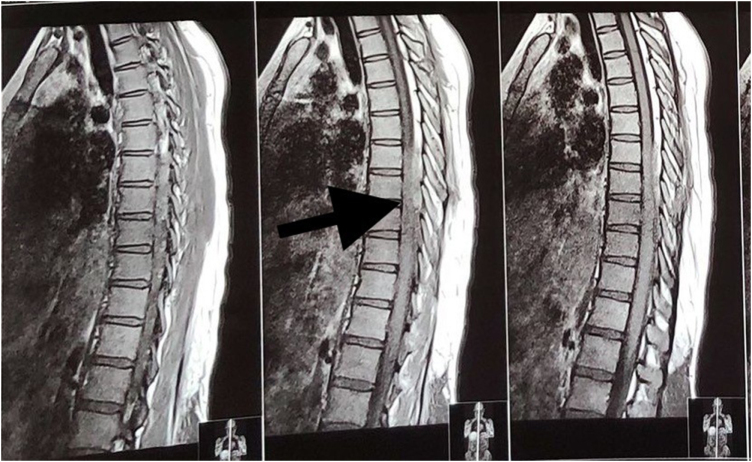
Sagittal T1-weighted MRI highlighting the extradural lesion compressing and deviating the spinal cord at T7–T9. Source: Author’s own image. Published with written informed consent from the patient.
